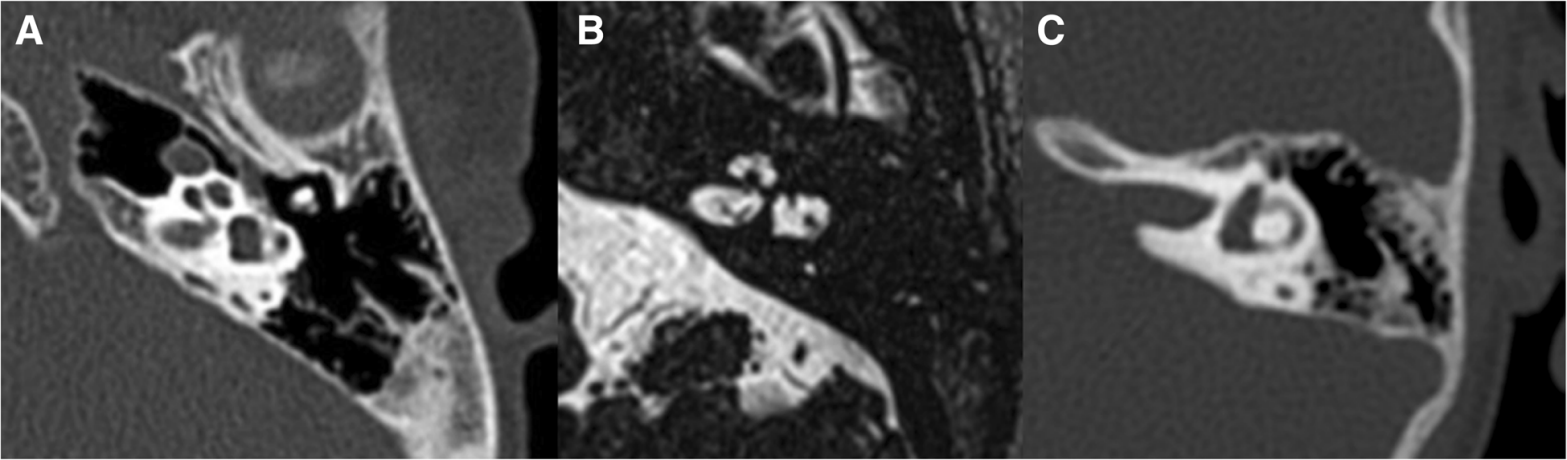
Temporal bone CT (a) and MRI (axial T2/3D) (b) from a 2-year-old Down syndrome patient at the level of the lateral semi-circular canal, revealing left hypoplastic bone island (less than 3 mm in greatest diameter). Axial temporal bone CT (c) at the same level of a normal 2-year-old boy for comparison
